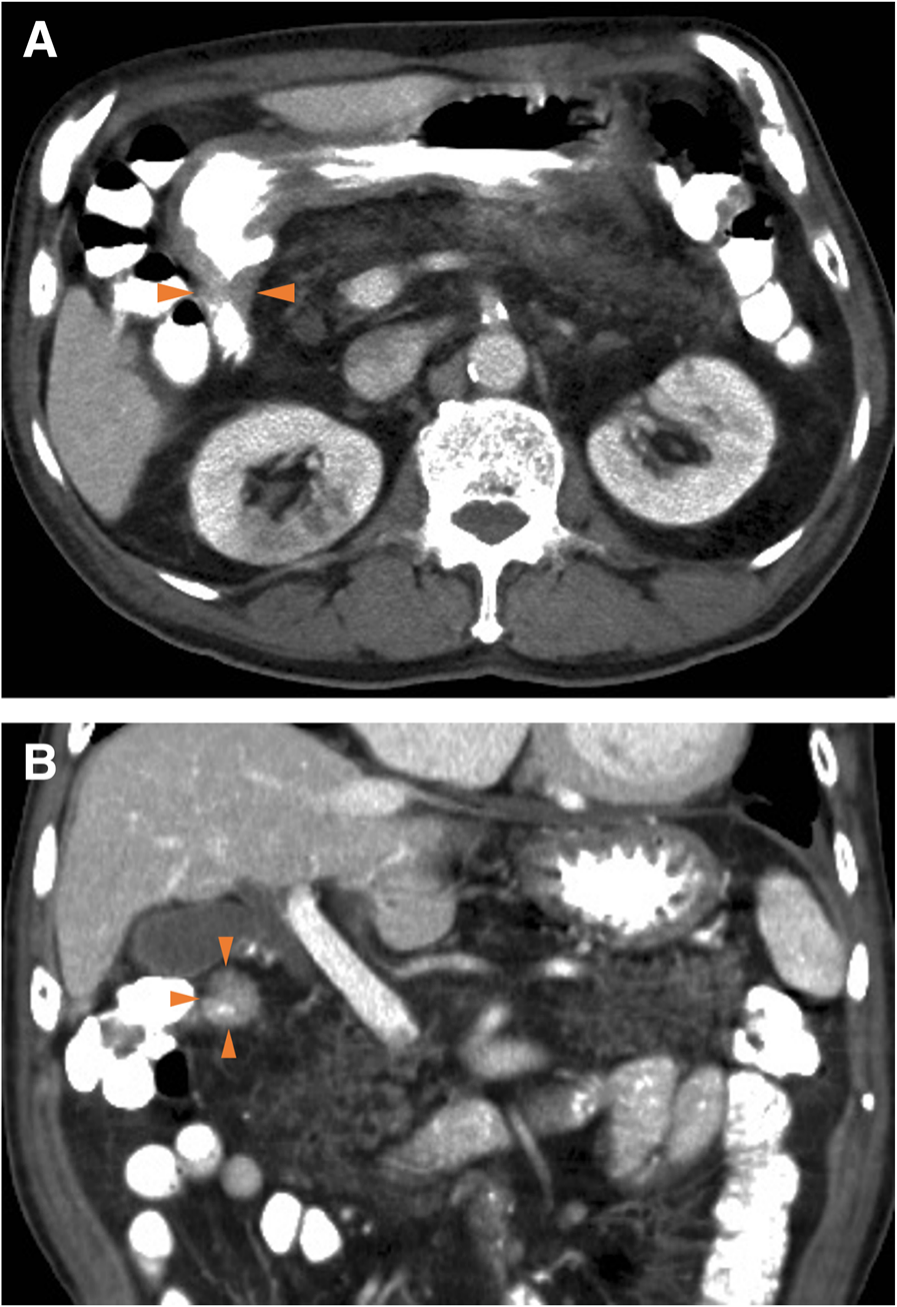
Enhanced multi-detector row computed tomography (MDCT) image showing the wall thickness in the duodenum in the transverse (a) and the coronal (b) planes. Arrow heads: wall thickness of the first part of the duodenum
